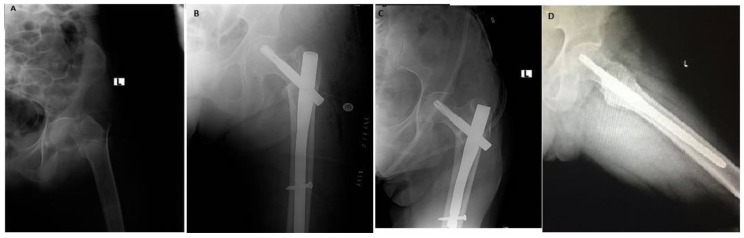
Type A3 fracture (A). Immediate postoperative AP radiograph showing a neck-shaft angle of 120° (B). 4 months follow up x-ray showing varus collapse with neck shaft angle of 115° (C). Lateral view at 4 months follow up (D).
